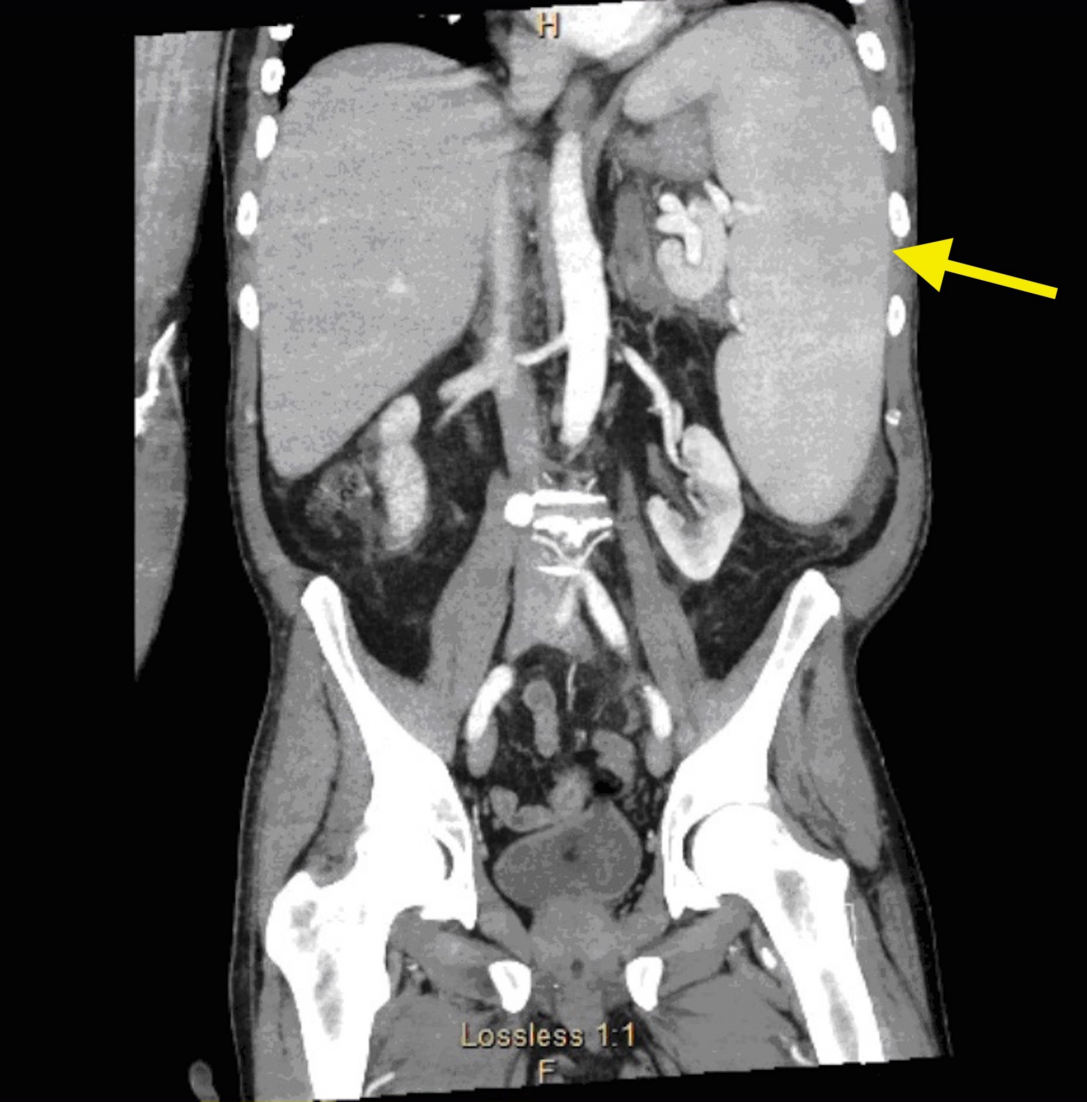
Computed tomography (CT) abdomen and pelvis demonstrating splenomegaly (yellow arrow).
